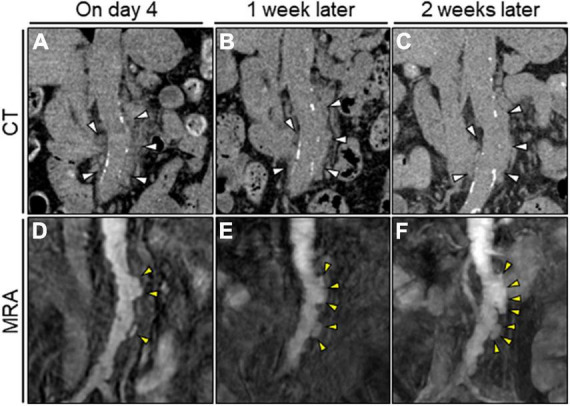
Serial unenhanced CT and MRA assessment of the abdominal aorta. (A–C) Serial unenhanced reconstructed coronal CT image showing no significant change in the periaortic mass of the abdominal aorta (white arrowheads). (D) Initial TS-MIP image of the original MRA image showing several asymmetric aortic dilatations in the abdominal aorta (yellow arrowheads). (D–F) Serial TS-MIP of the original MRA image showing rapid aortic expansion with multilobular protrusion of the abdominal aorta to the left side (yellow arrowheads). CT, computed tomography; MRA, magnetic resonance angiography; TS-MIP, thin-slab maximum intensity projection.
